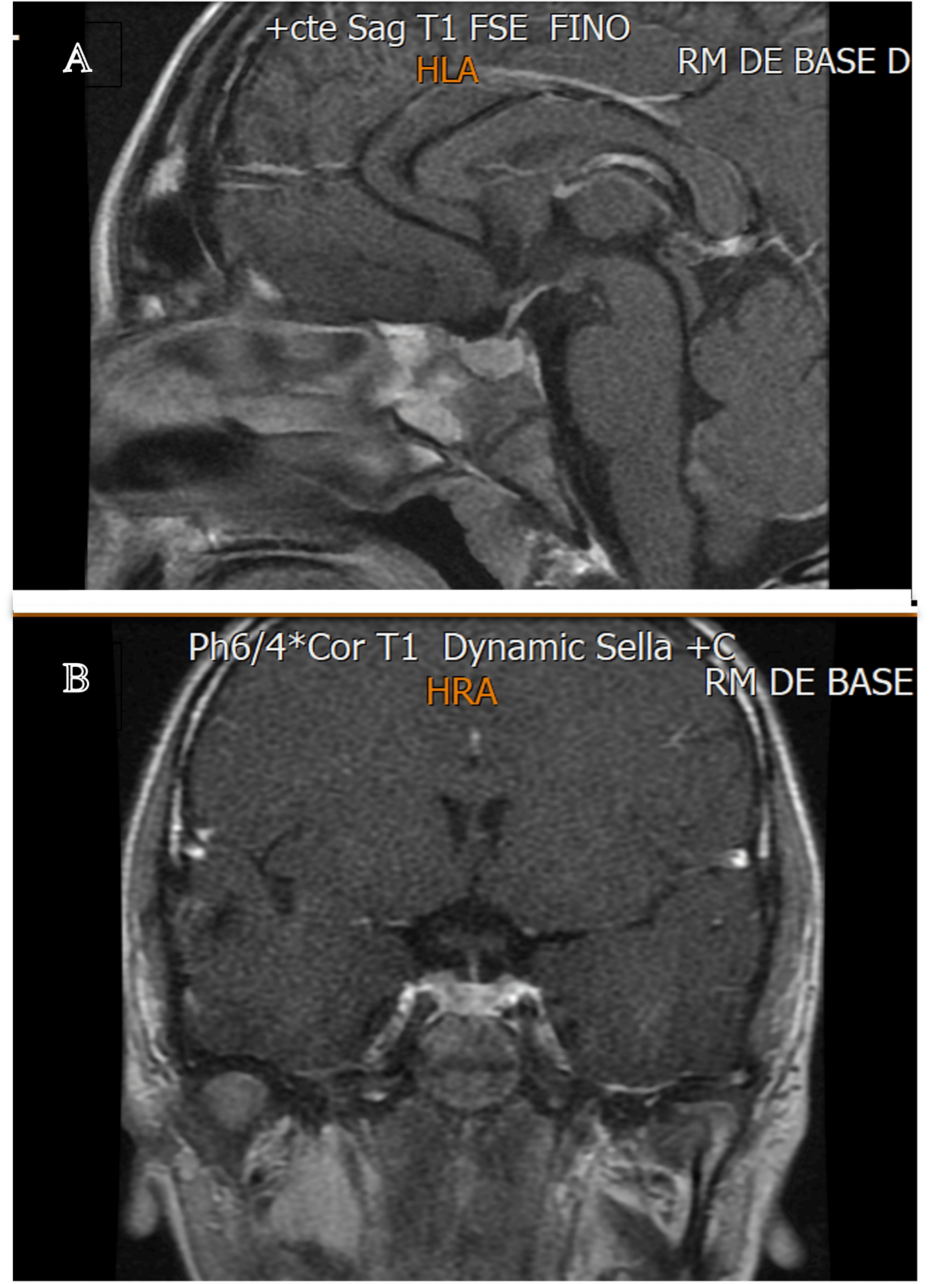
The MRI of the pituitary gland obtained three months after treatment showed regression of the enlargement and restoration of normal size (A) sagittal view; (B) coronal view
